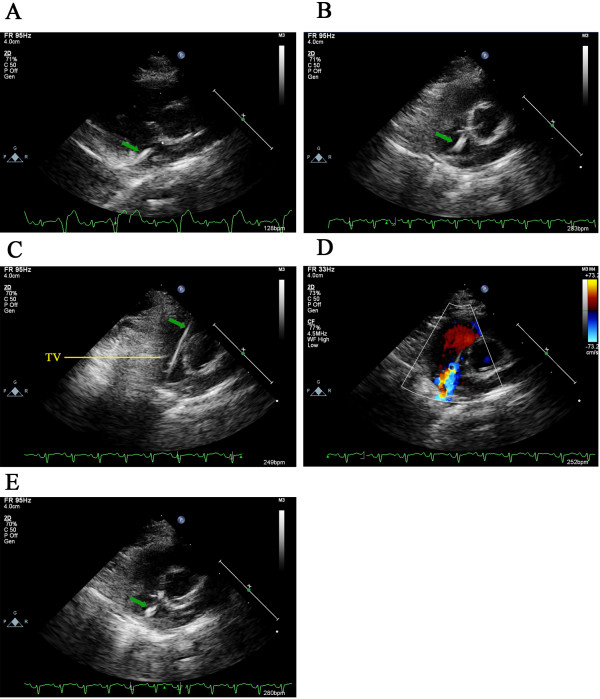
The process of right-heart catheterization under echocardiographic guidance. Echocardiography with an aortic short axis view was used to visualize the position of the catheter system. The catheter system was visualized entering the right atrium (A). The guide wire was slowly backed out to connect a pressure transducer to measure pressures in the right atrium. The pressure transducer was removed to allow introduction of the guide wire into the catheter system again. Adjust the direction of the guide wire to advance the catheter system pointing to the tricuspid valve (B) and then getting through it (C). The guide wire inside the catheter was then used to damage the tricuspid valve. Color Doppler was in action to visualize tricuspid valve regurgitation (D). The peak velocity was then evaluated by Pulsed Doppler. The catheter system was then repositioned at the entrance of the right atrium (E). The guide wire was removed and a pressure transducer connected to measure the pressure of the right atrium after valvular impairment.
